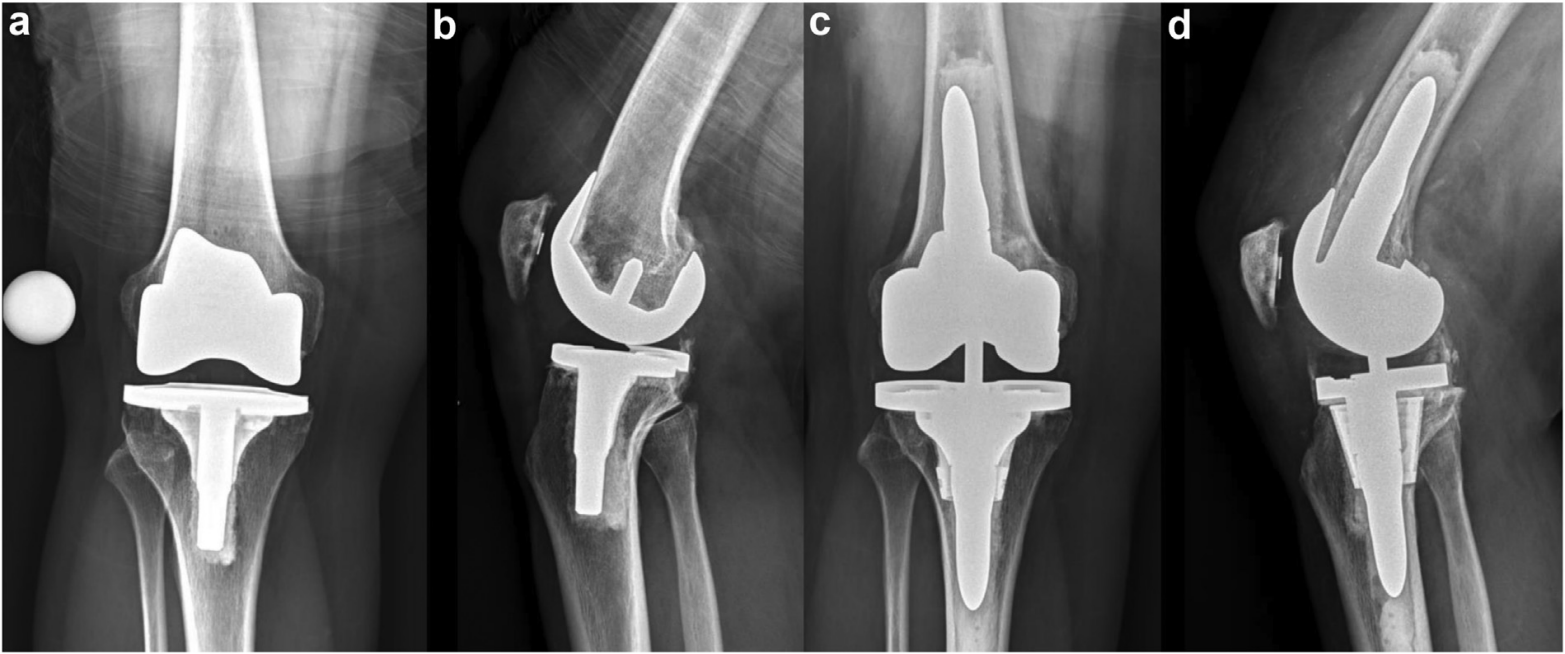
(a) Anteroposterior and (b) lateral preoperative radiographs of an unstable TKA. There is slight extension of the femoral component with overresection of the posterior femoral condyles and increased posterior slope on the tibial component, which contributed to the flexion instability in this patient. (c) Anteroposterior and (d) lateral postoperative radiographs depicting a balanced revision TKA with (3 degree) flexion of the femoral component and posterior femur augmentation to restore the lost femoral posterior condylar offset. Short revision IM stems with a tibial metaphyseal cone is characteristic of robotic assisted revision TKA.
